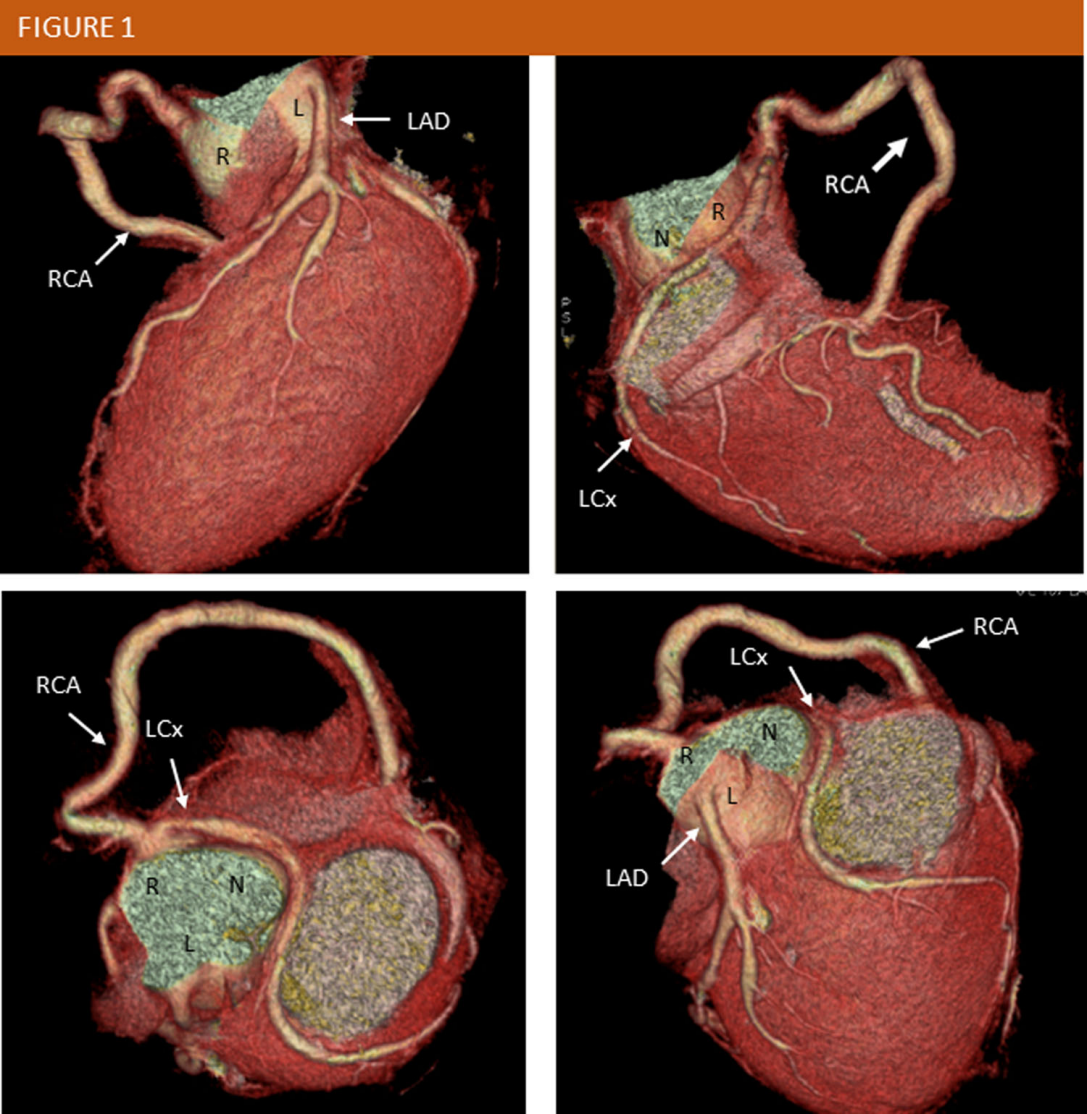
CCTA volume rendering images of anomalous origin of left circumflex artery from the right coronary sinus with shared ostia. L: left coronary sinus; N: non coronary sinus; R: right coronary sinus. CCTA, coronary computed tomography angiography; LAD, left anterior descending; LCx, left circumflex artery; RCA, right coronary artery.
